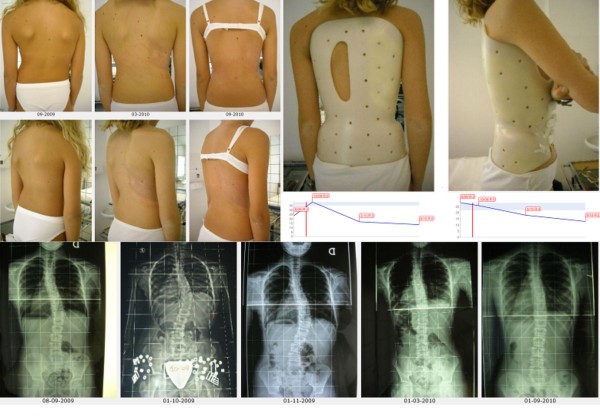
Third case report: adolescent thoraco-lumbar scoliosis patient rapidly progressing still in treatment. On the left: posterior (first line), and sagittal (second line) aesthetics. On the right: the brace used, and the graph with obtained results. On the bottom line: all x-rays of this case report of a patient still in treatment. G.B. presented in september 2009, 10 years old, with a first x-ray showing a thoracic left, thoraco-lumbar right curve of 28°-24° (Risser 0): parents stated that they had seen their daugther worsening in the 15 days span between the x-ray and the medical evaluation. At first a SpineCor brace has been prescribed but the x-ray within brace showed such a bad situation (14°-30°) to suggest to re-evaluate a radiograph without the brace: scoliosis was rapidly worsening (26°-39°). We decided to move to a SPoRT brace and SEAS exercises (twice a week, 45' per session): Sibilla 23 hours per day. In 6 months, while growing 6 cm. (from 145 to 151), she corrected to 17°-18°, and in 6 more months wearing the brace 22 hurs per day, she arrived to 13°-14°, during an height increase of other 6 cm. (from 151 to 157). Now she continues to be Risser 0, and is wearing the brace 21 hours per day.
